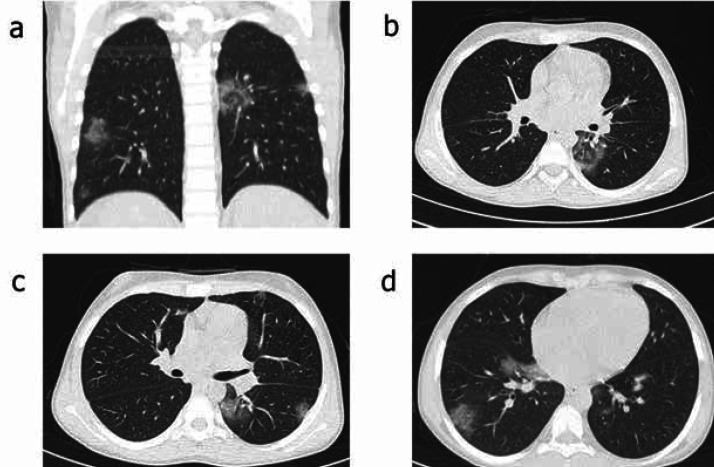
The chest CT scan without contrast in a 14-year-old boy with chronic liver cirrhosis. There are bilateral multifocal sub-pleural ground-glass opacities with mild pleural effusion highly suggestive for COVID-19
